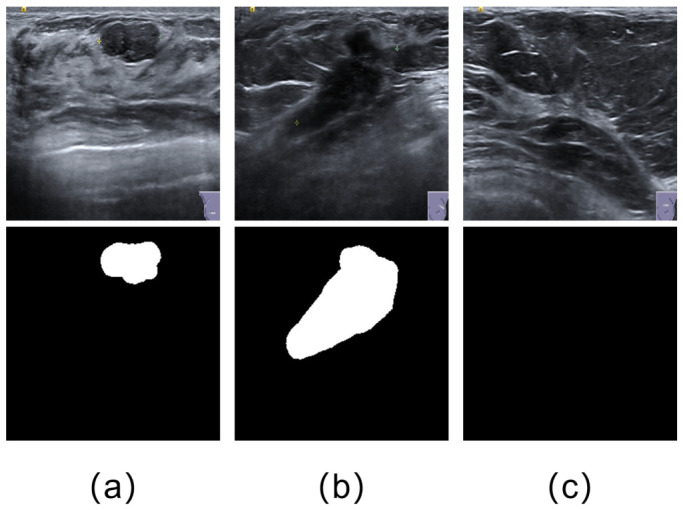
Sample images from the BUS-UCLM dataset. The first row presents the original ultrasound images, while the second row shows the binary ground-truth masks used in this study, where white denotes the lesion foreground and black denotes the background. (a) benign case and its corresponding binary mask, (b) malignant case and its corresponding binary mask, and (c) normal breast tissue without lesions and its corresponding binary mask.
